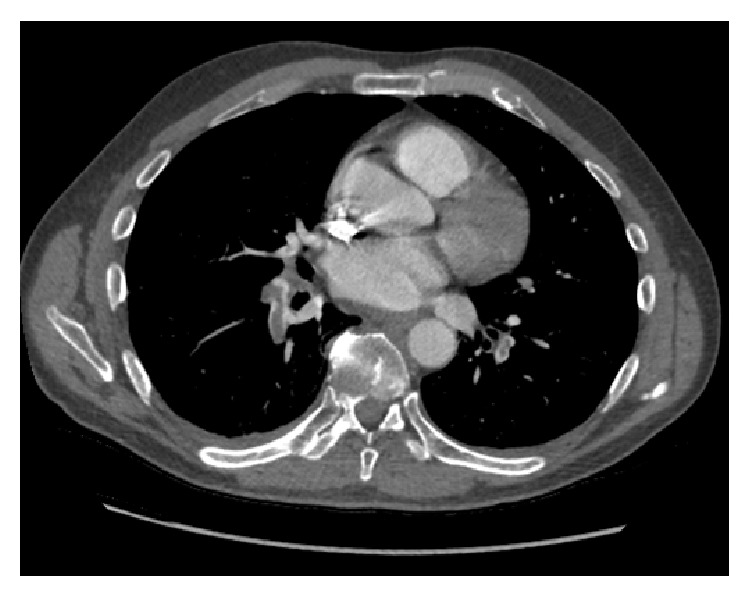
Chest CT showing bilateral filling defects involving the right and left main pulmonary arteries with extension to the segmental branches of the right middle and bilateral lower lobes.
